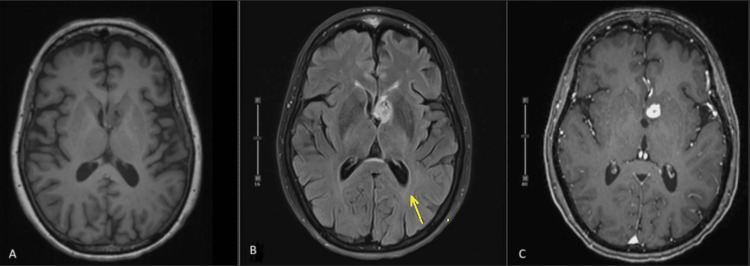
Post-treatment MRI Axial T1 images without contrast. (a) T2 FLARE (b) contrast-enhanced T1 (c) images of the brain post-chemoradiotherapy and subtotal resection. Images demonstrate a residually enhancing lesion along the interventricular septum. The ventricular dilatation and associated transependymal edema have resolved (yellow arrow).
